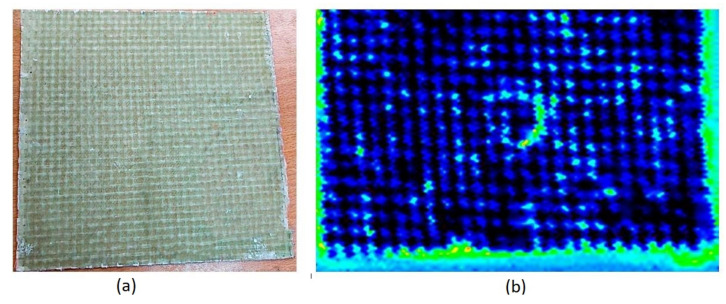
GFRP panel (a) and C-Scan representation of the ultrasonic inspection (b), showing damage caused by repeated slamming impacts.
